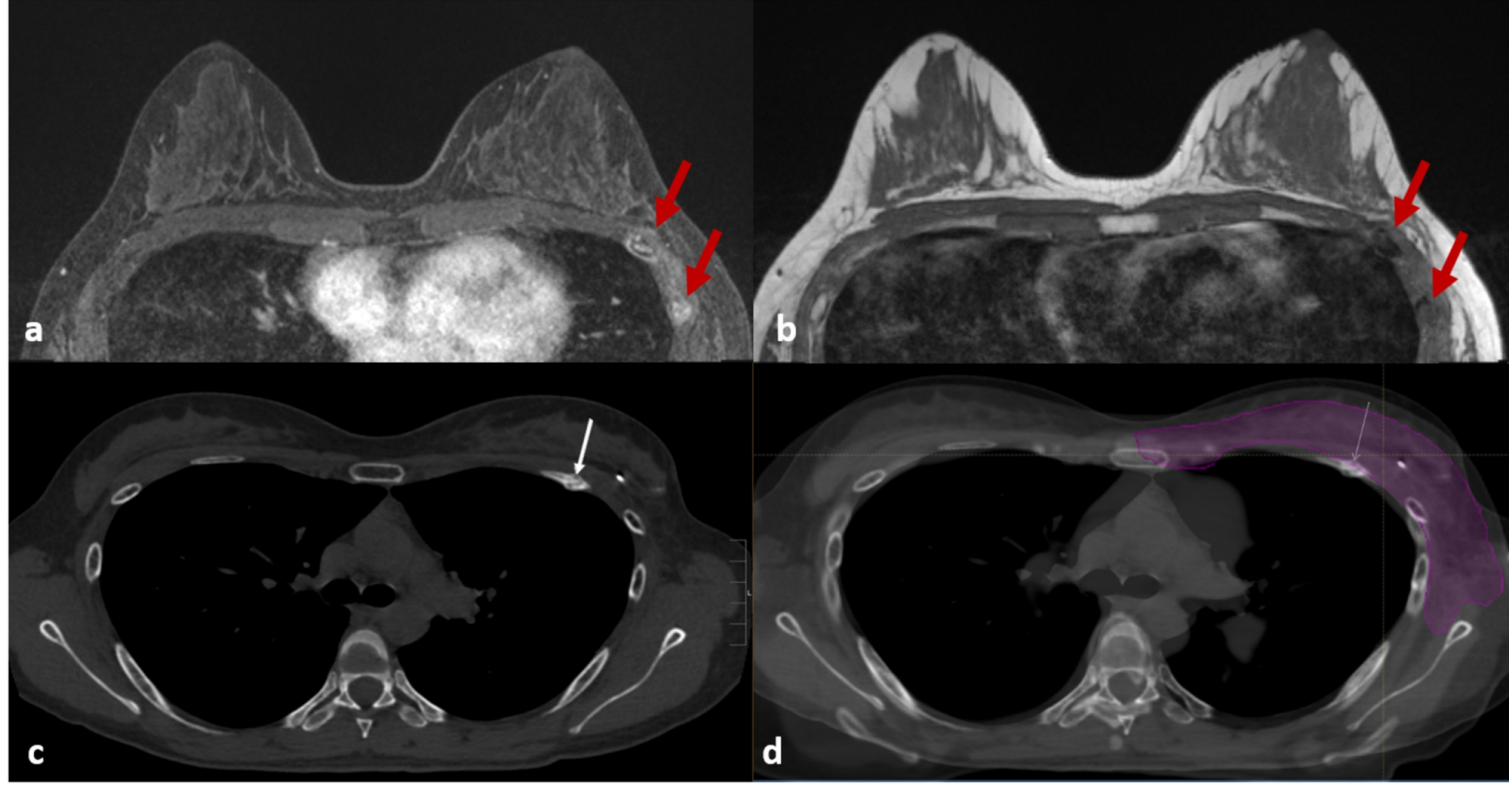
Patient ribs 3, 4, and 5. MRI of the breasts, (a) axial T1 fat saturation post-contrast, (b) axial T1 non-fat saturation; demonstrated focal areas of T1 hypointensity with enhancement of the ribs without adjacent soft tissue abnormality (red arrows) located immediately posterior to the lumpectomy bed and left axilla. Axial CT of the breast, (c) using bone windows demonstrates non-displaced left-sided anterior rib fractures involving the third through fifth ribs (white arrow). CT transposed onto axial slice (d) patient treatment plan; magenta representing the 95% isodose line of the prescription dose.
